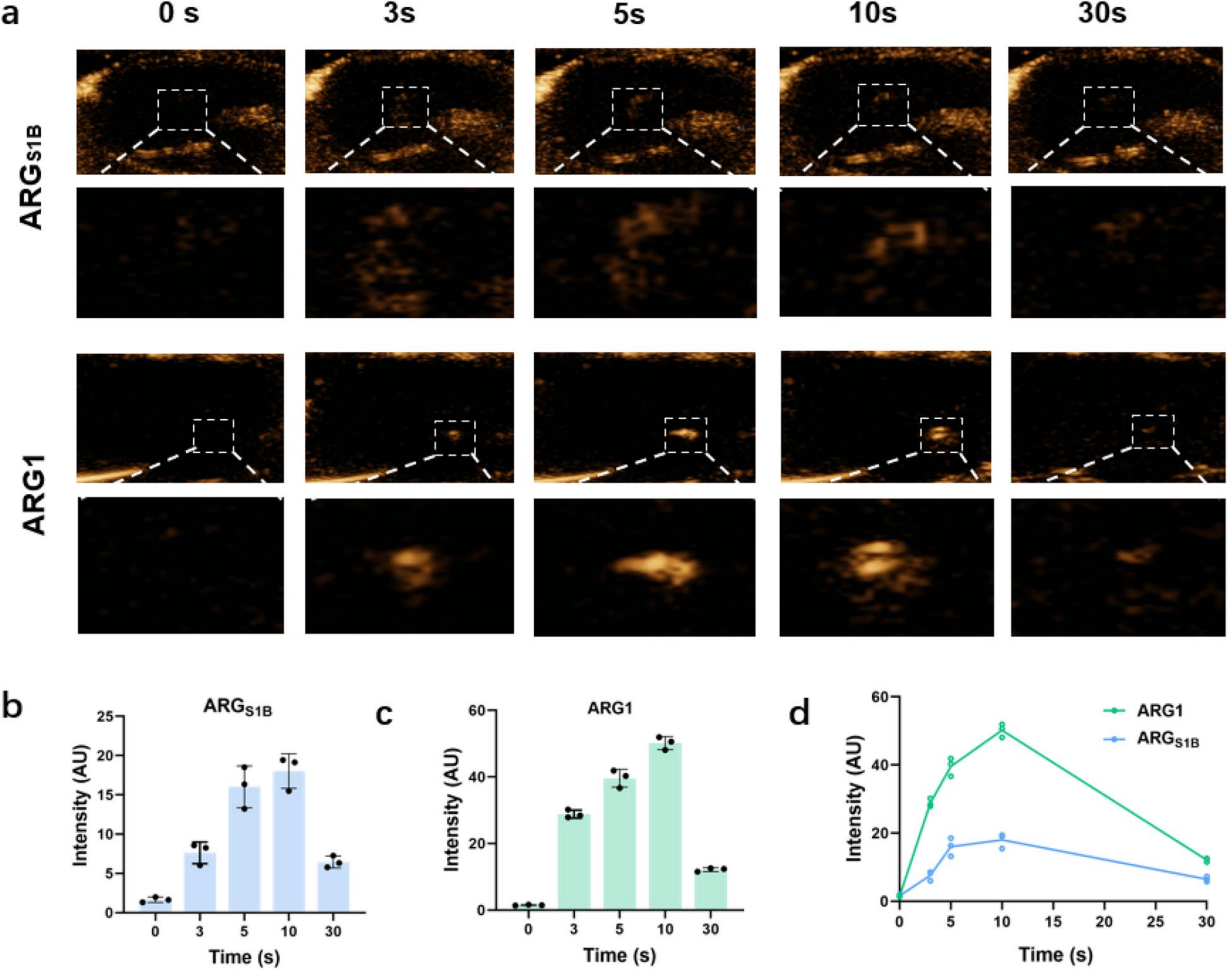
Characterization of ARG_S1B_ in vivo. a The ultrasound contrast images of the liver received with ARG_S1B_ or ARG1 at different time points, OD_500_ = 3.0. b, c Contrast signal intensity in the liver (white-dotted rectangular regions). d Mean ultrasound contrast from ARG1 and ARG_S1B_ at different injection time. Error bars representing mean ± SD for *N* = 3 replicates
